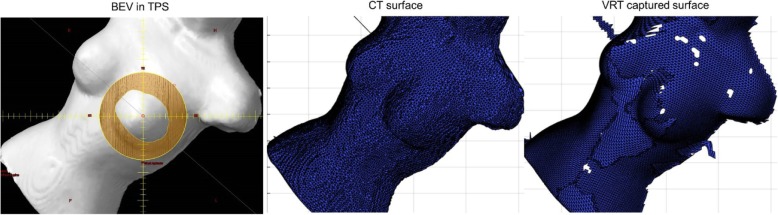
An example of comparison of BEV in TPS, reconstructed CT surface, and AlignRT (VRT) captured surface
